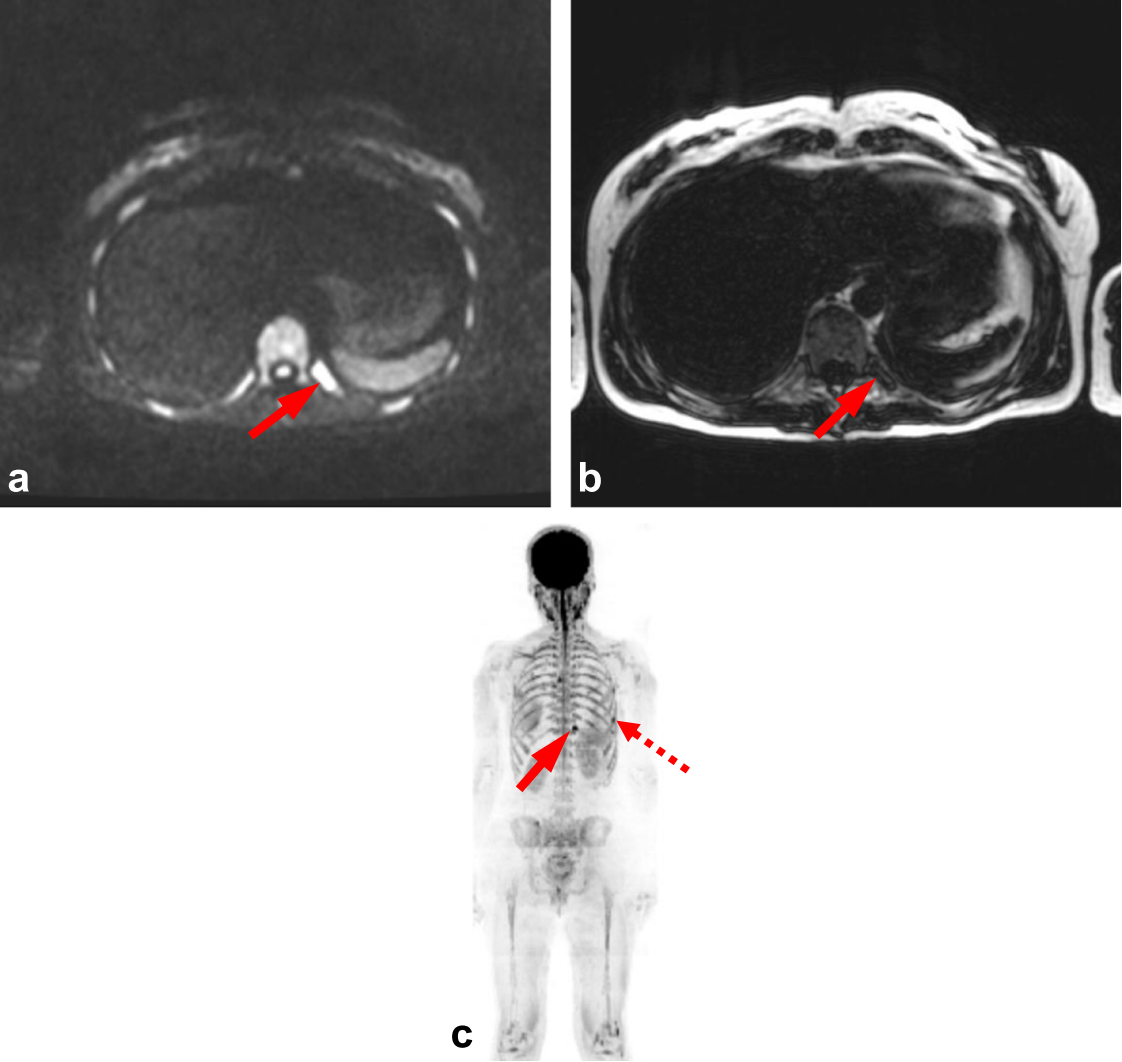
Focal lesion of ribs. Observer score variation within the ribs due to focal lesion (arrows) superimposed on diffuse marrow infiltration, shown by (a) axial b900 DW MRI, b axial fat-only Dixon and (c) b900 maximum intensity projection (MIP). Diffuse marrow infiltration results in high diffusion signal throughout ribs (a, c) which conceals superimposed focal lesion (arrow a). Fat only Dixon and MIP facilitate focal lesion differentiation (arrows b, c). Note MIP also demonstrates second focal lesion (dashed arrow c)
